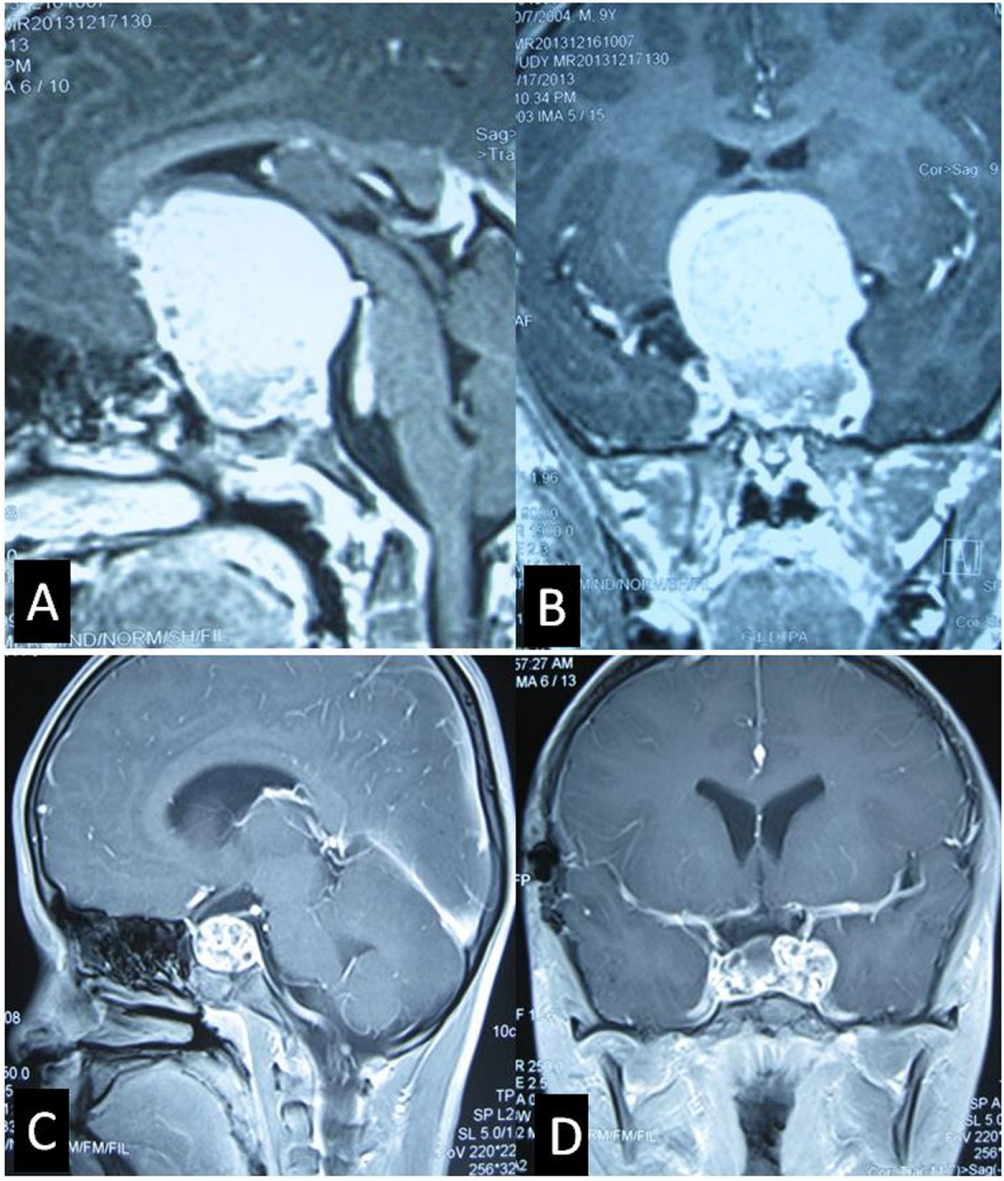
Growth pattern of Intrasellar stalk CPs. (A and B) Sagittal (A) and Coronal (B) postcontrast T1-weighted MR images showing a giant solid Intrasellar stalk CP grows in vertical direction and pushes the hypothalamus upward, at the same time, sella turcica is obviously much enlarged by the tumor. (C and D) Sagittal (C) and Coronal (D) postcontrast T1-weighted MR images showing a Intrasellar stalk CP grows laterally into the left cavernous sinus.
